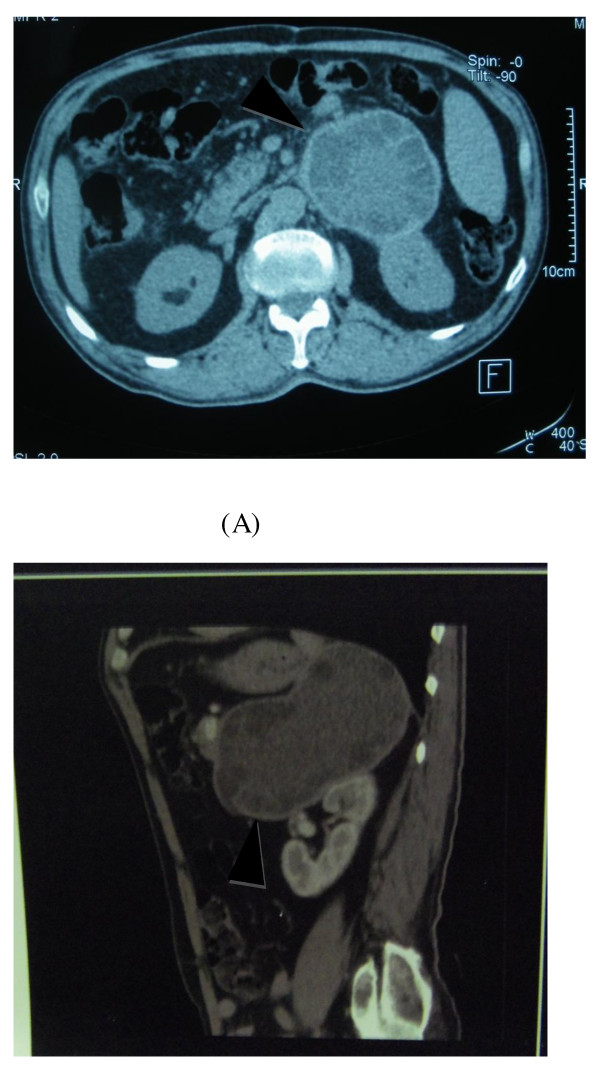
Type III hydatid cyst. Computed tomography scans show daughter cysts filing the lesion, which has a honeycomb appearance.
